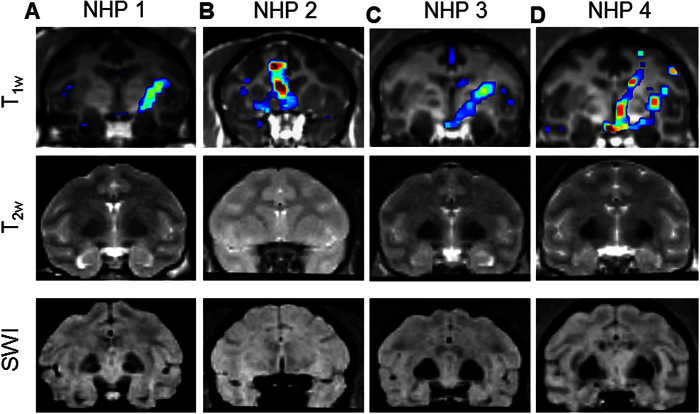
Safety assessments in the MRI. (A) NHP 1 targeting putamen at 275 kPa. (B) NHP 2 targeting caudate nucleus and putamen at 400 kPa. (C) NHP 3 targeting putamen at 450 kPa. (D) NHP 4 targeting caudate nucleus and putamen at 600 kPa. No edema or hemorrhage was detected in any of the cases. 1^st^ row: contrast enhancement of the BBB opening overlaying onto the post-Gd T_1w_ imaging; 2^nd^ row: T_2w_ imaging for edema; 3^rd^ row: susceptibility-weighted imaging (SWI) for hemorrhage.
